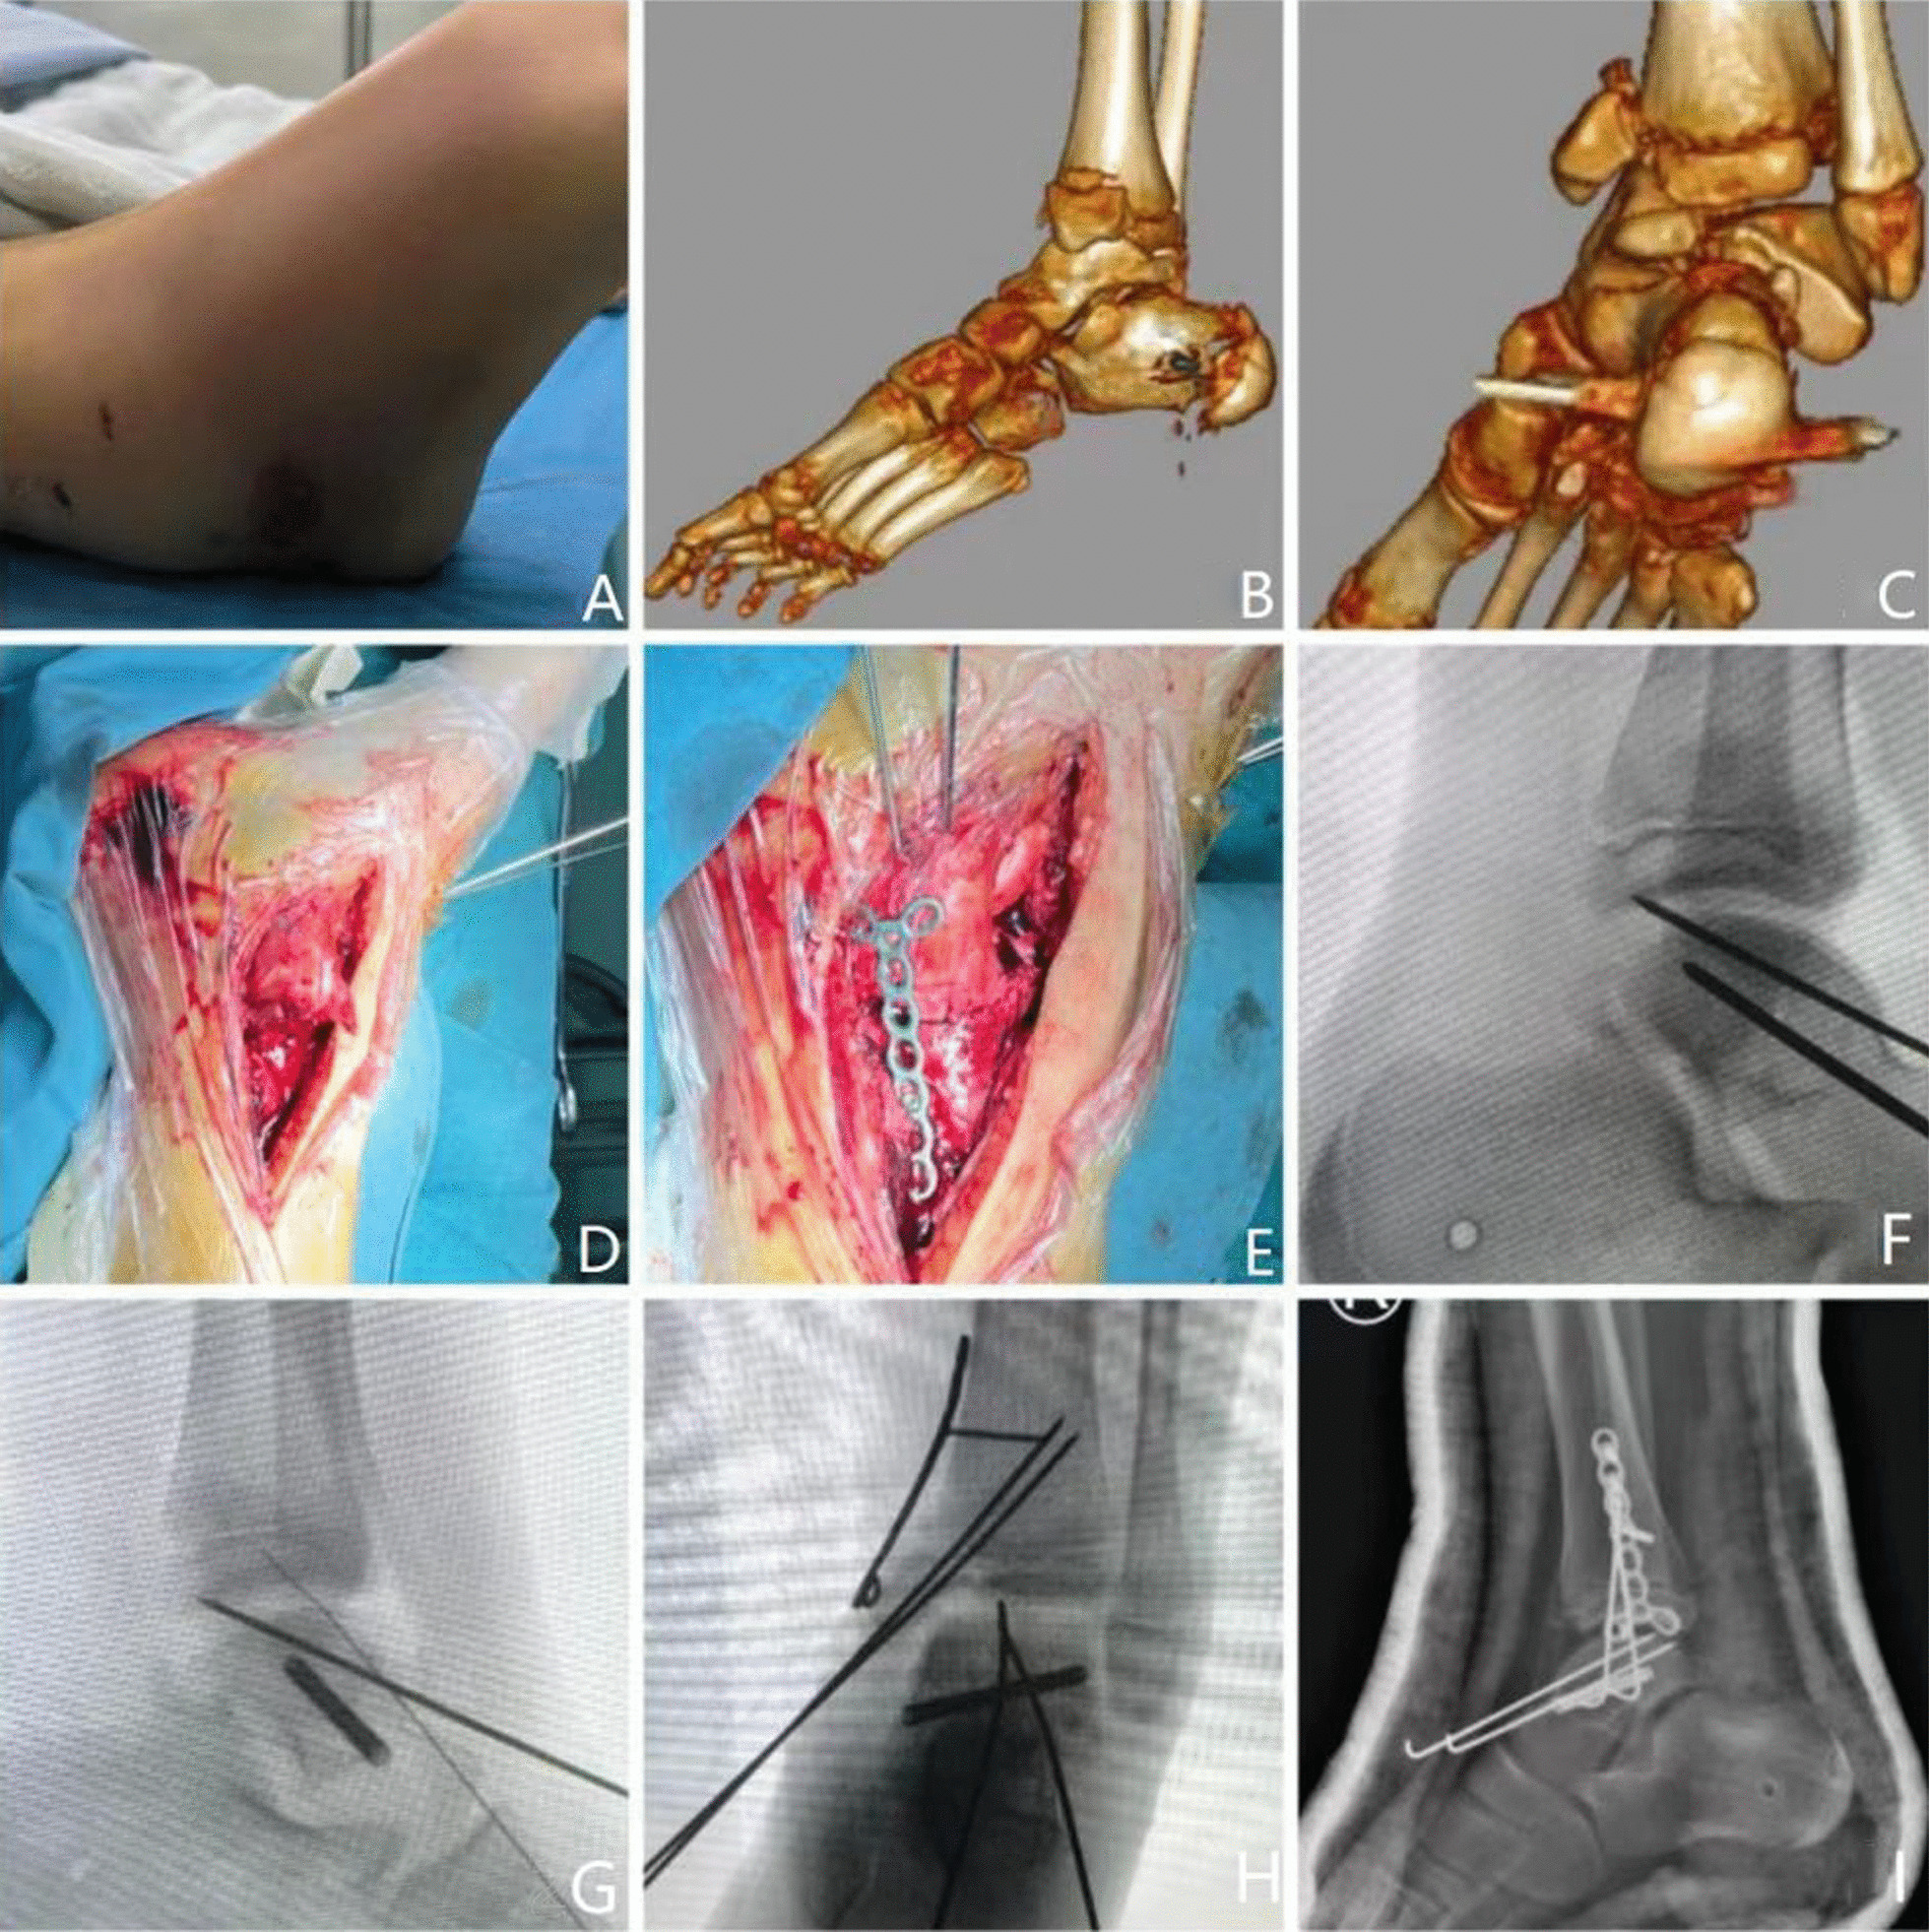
A 9-year-old female patient presented with a right talar fracture caused by a high fall injury. The swelling of the ankle joint gradually disappeared after removing the calcaneal traction device before the operation (A). The color film of ankle joint trauma before the operation (B, C) showed a type II fracture of the right talus neck. Intraoperative reduction under direct vision (D, E), open reduction and cannulated screw and plate and Kirschner wire fixation were performed. Intraoperative C-arm fluoroscopic ankle joint X-ray (F, G, H) showed good reduction in the fracture. At 2 months after the operation, the patient still received plaster external fixation, and the X-ray (I) of the ankle joint showed that the joint space was good, the fracture position was well aligned, and there was no obvious sign of avascular necrosis of the talus neck. The postoperative AOFAS score was 95
